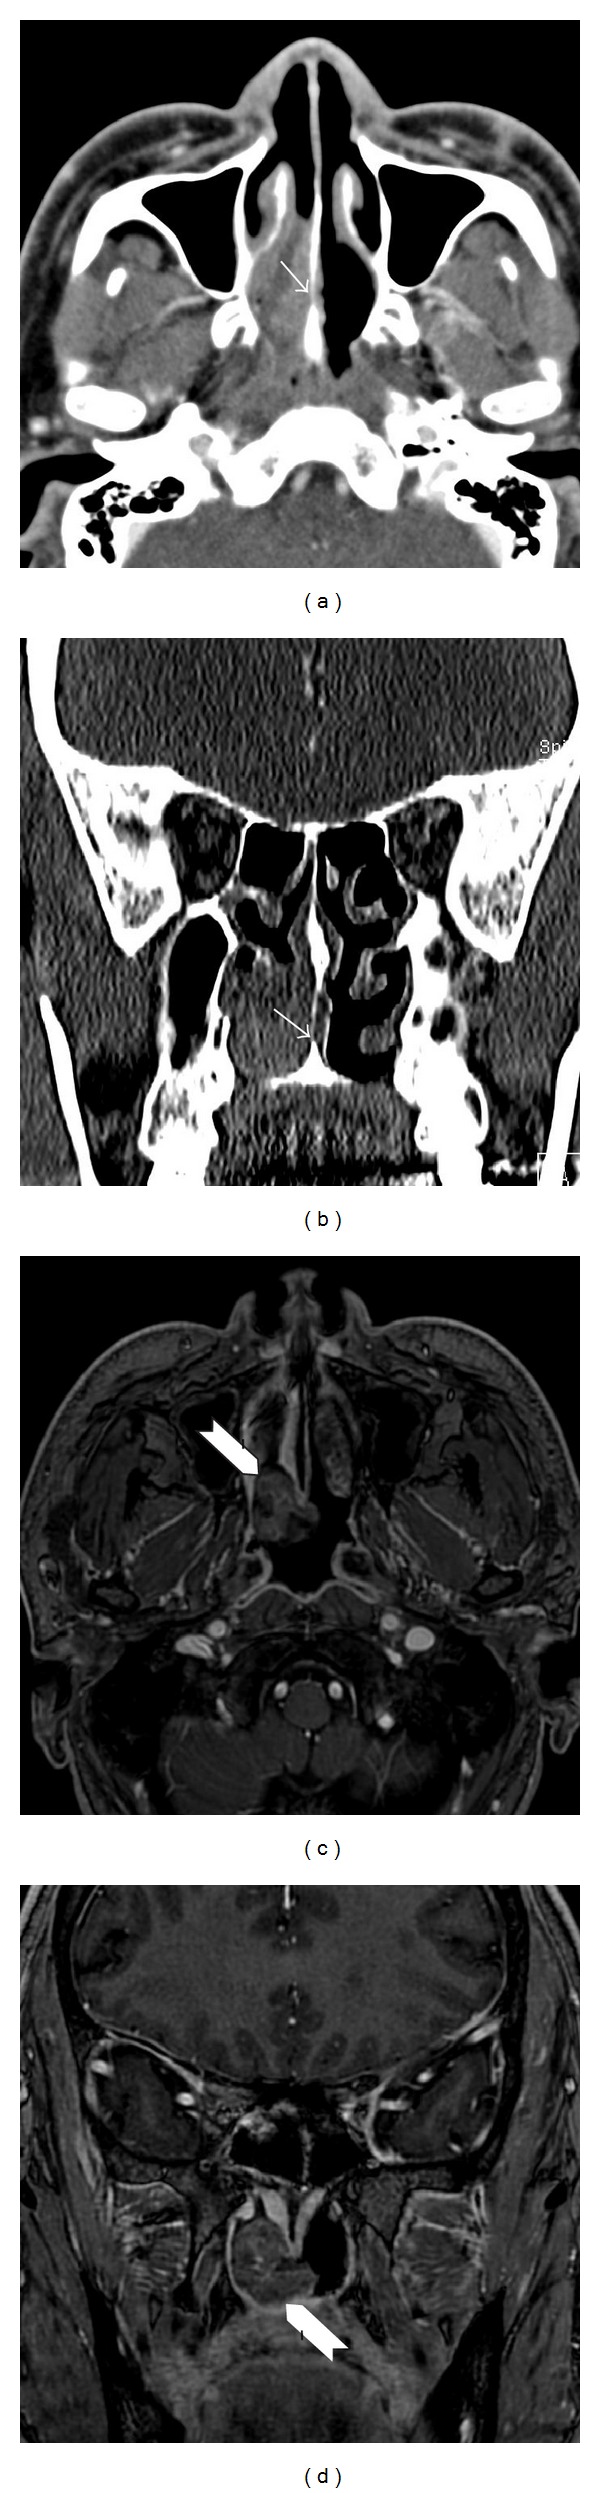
Axial (a) and coronal (b) images of paranasal sinus computed tomography. The mass shows a heterogeneous density between the nasal septum and lateral nasal wall, with erosion of the septal bone (arrow). Axial (c) and coronal (d) images of paranasal sinus T1-gadolinium-enhanced magnetic resonance imaging. A 2.5 × 1.5 cm sized, gadolinium-enhanced fungating mass with internal hemorrhage, and vascularity (arrowhead) can be seen between the right choana and lateral wall. The lesion is well demarcated with smooth margins.
